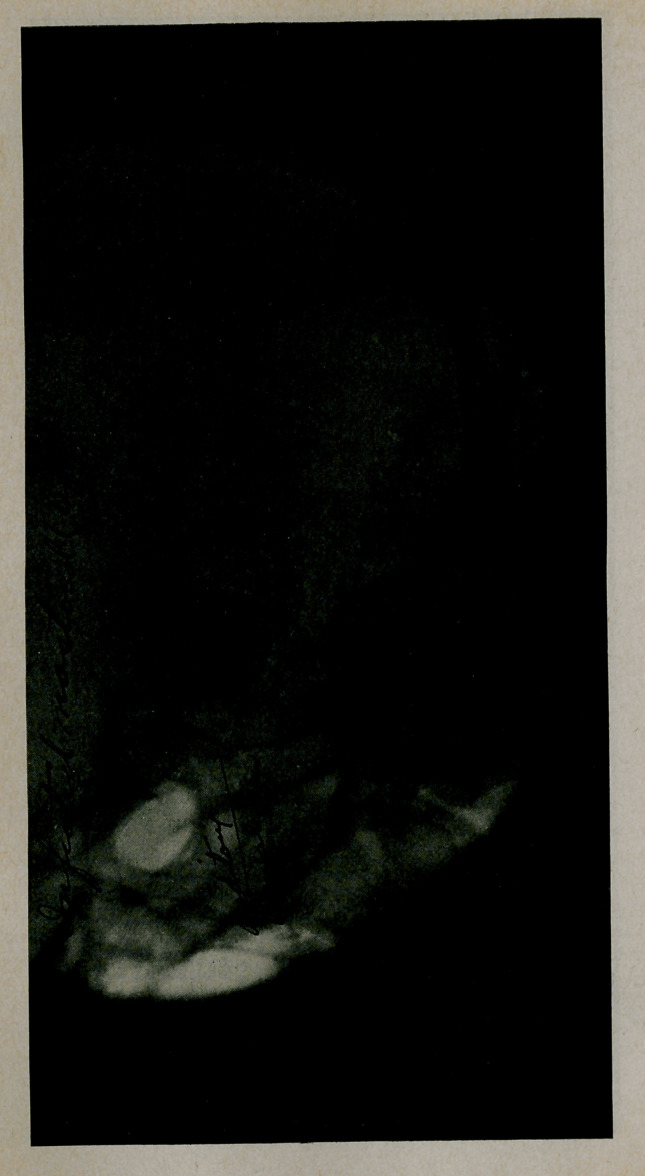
# The Value of Radiographs in the Diagnosis of Mastoditis
